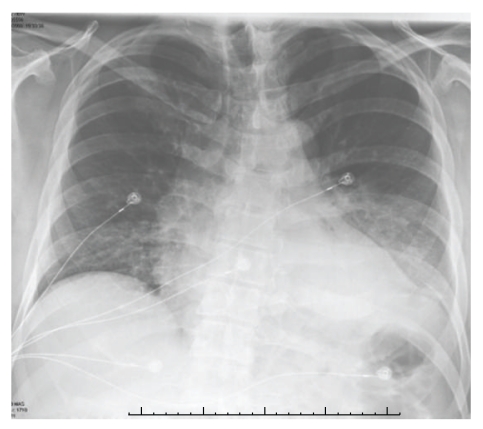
Chest X-ray on day 1 showing bilateral basal infiltration.
